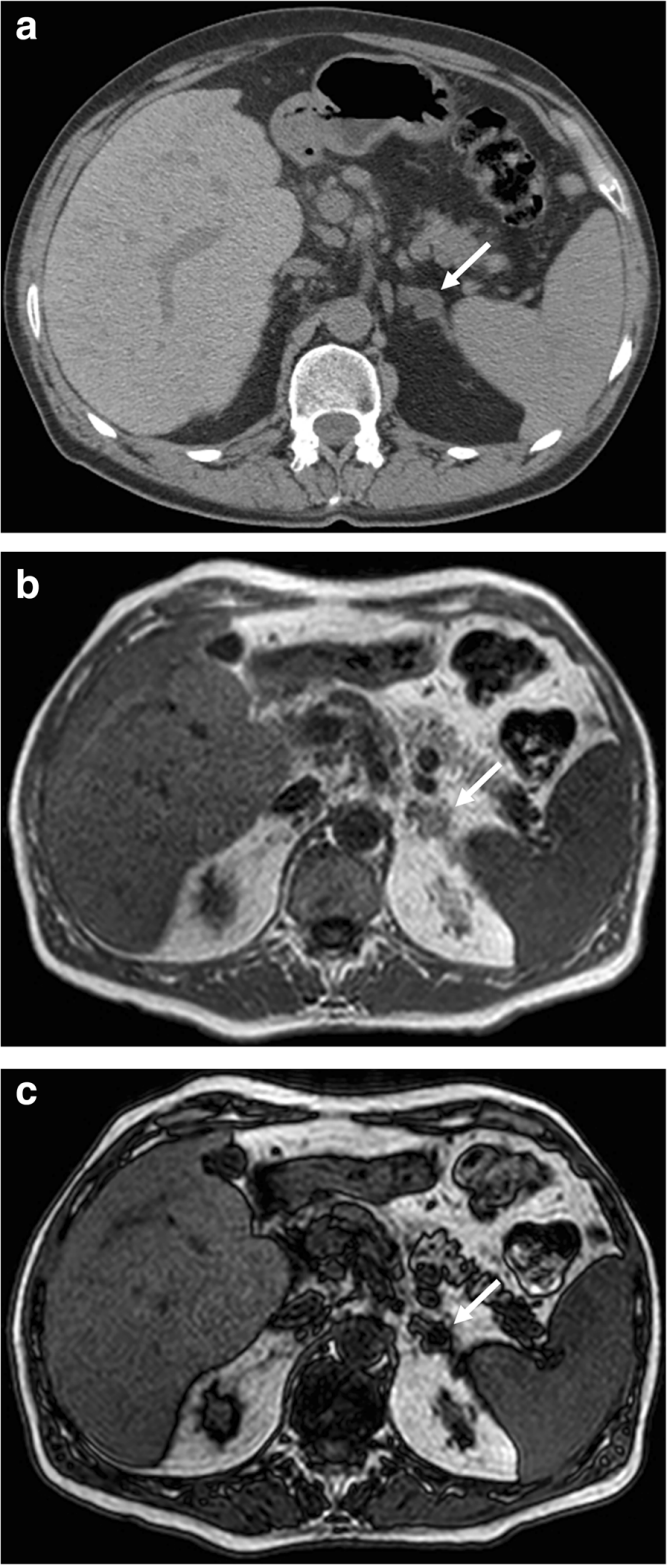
Adrenal adenoma of a 45-year-old woman. Unenhanced CT (a) shows a left adrenal mass (arrow) with lower attenuation values than 10 HU. Axial T1-weighted in-phase (b) and out-of-phase (c) gradient recalled echo MR images show prominent loss of signal on out-of-phase
